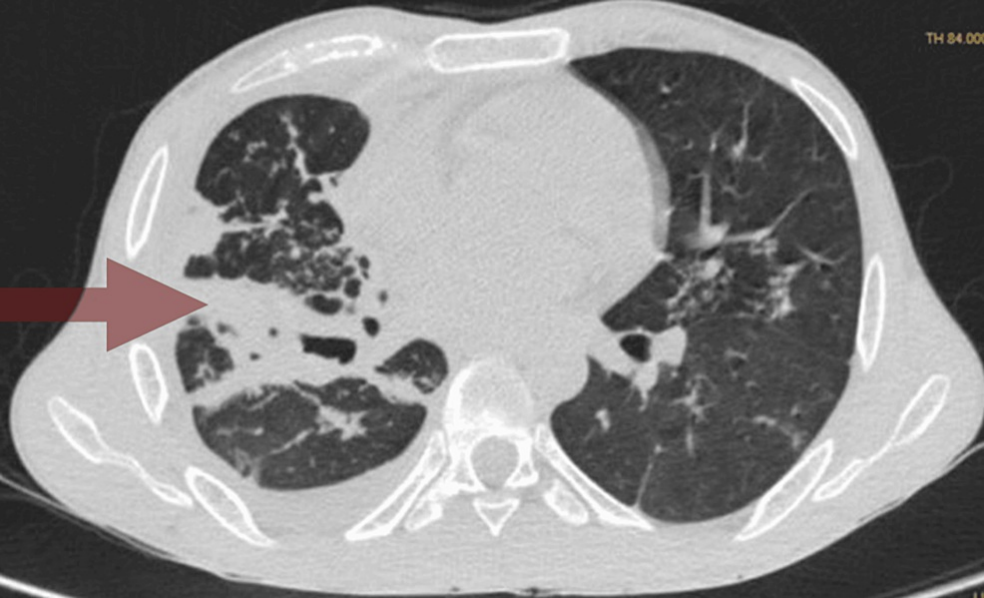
HRCT thorax in axial view showing ground glass opacities with consolidation (red arrow) in the right upper lobe HRCT: High-resolution computed tomography
